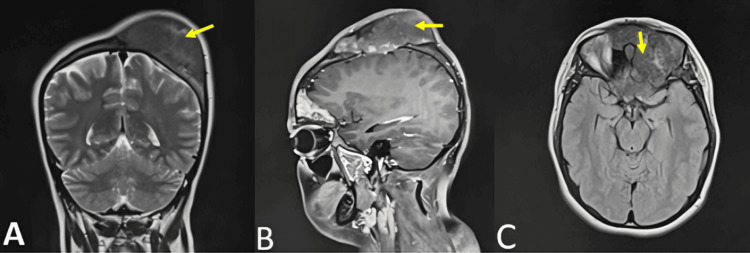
MRI showing a mass with relatively well-defined margins and slight lobulations, centered on the frontal bone and extending to the left parietal, ethmoidal, and sphenoidal bones (arrow). It measures approximately 7 x 7.5 x 6 cm (T x AP x CC). (A) Coronal section on T2-weighted image. (B) Sagittal section on T1-weighted image after gadolinium injection. (C) Axial section on FLAIR sequence. FLAIR, fluid-attenuated inversion recovery
